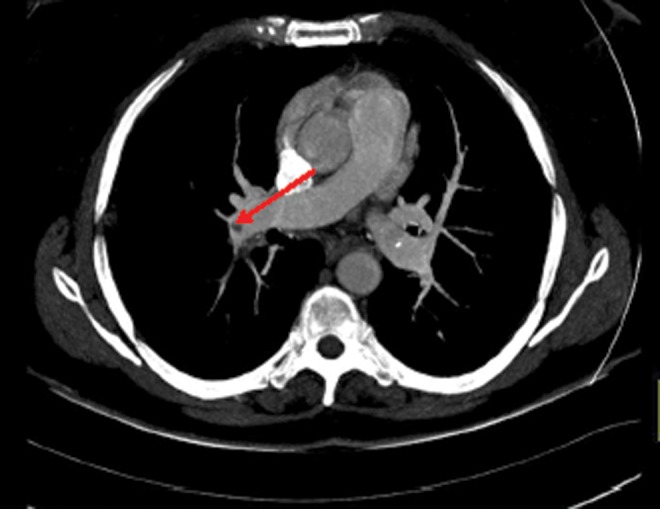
Patient 1: Axial image of computed tomography (CT) pulmonary angiogram with filling defect in right pulmonary artery (*arrow*) indicating thrombus.
